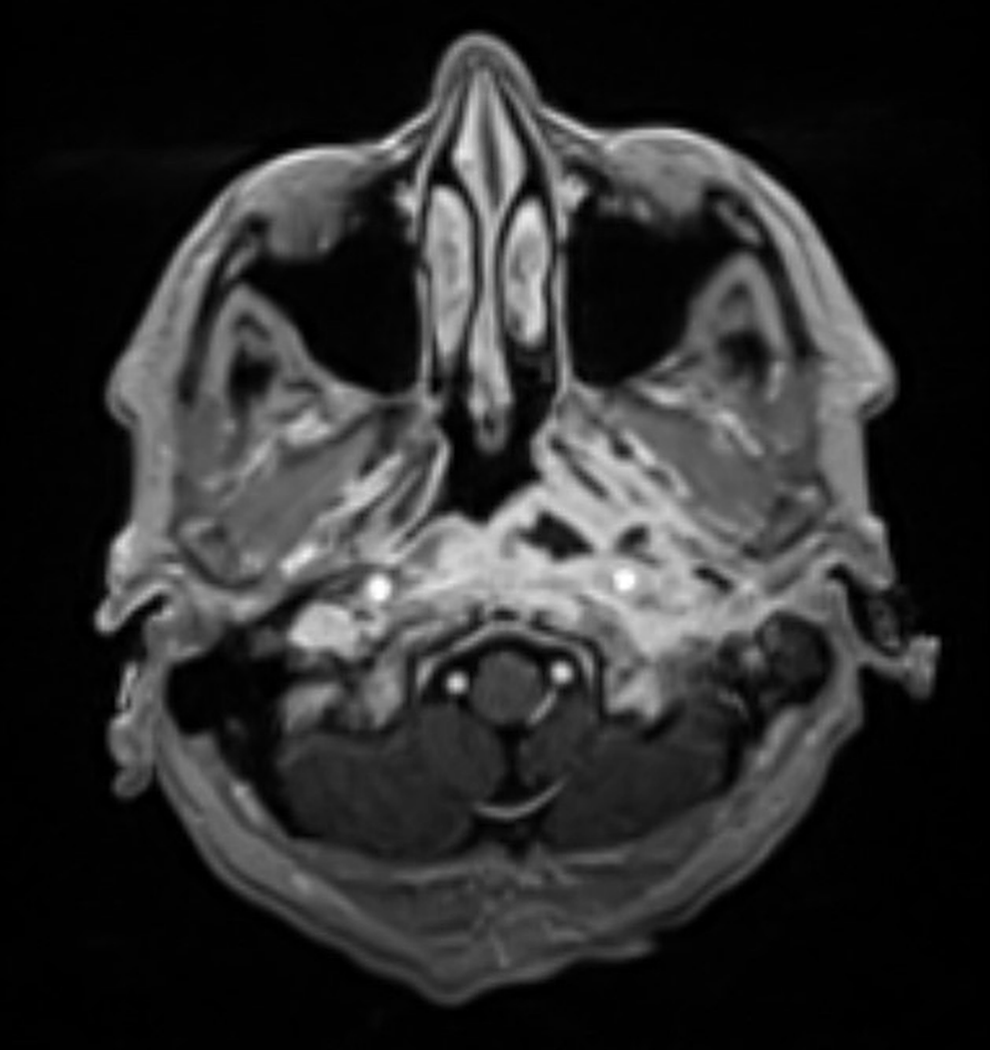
MRI examination at the start of treatment (TSBO)
